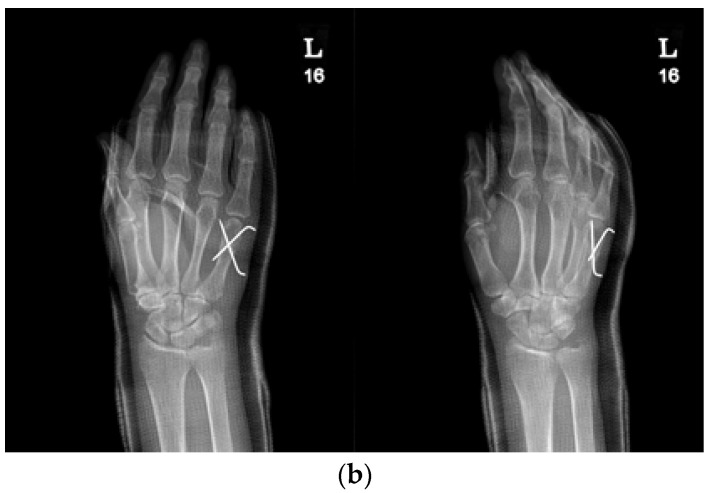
(a) Radiograph of a 70-year-old female patient with displaced little-finger metacarpal neck fracture of the left hand. (b) Radiograph of a 70-year-old female patient who underwent the KP method using two K-wires.
